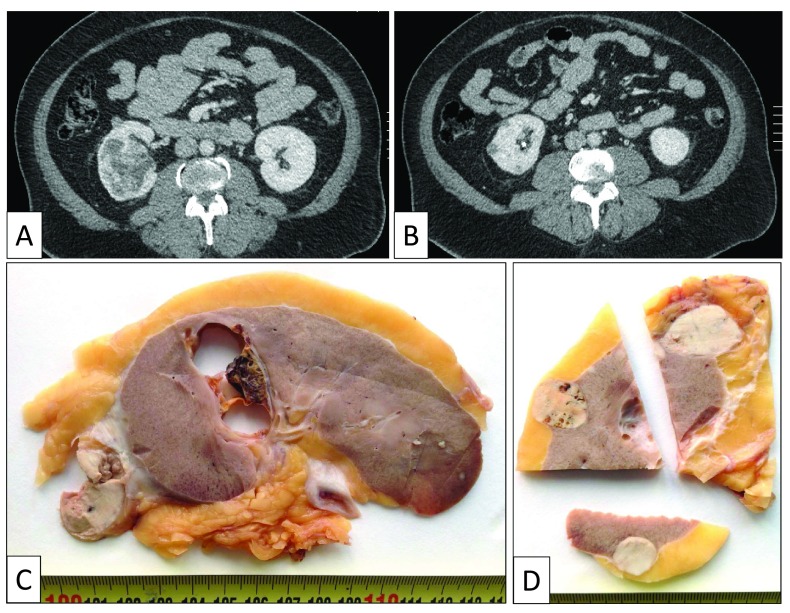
CT scans show multiple tumors in the right kidney (
